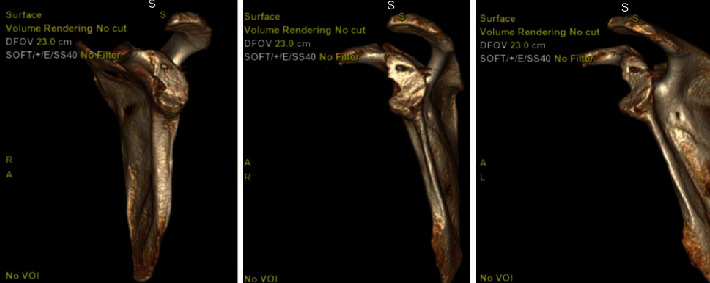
CT scan left shoulder with 3D reconstruction and humeral subtraction (case 2). A large glenoid rim fracture fragment is seen involving approximately 20% of the articular surface.
